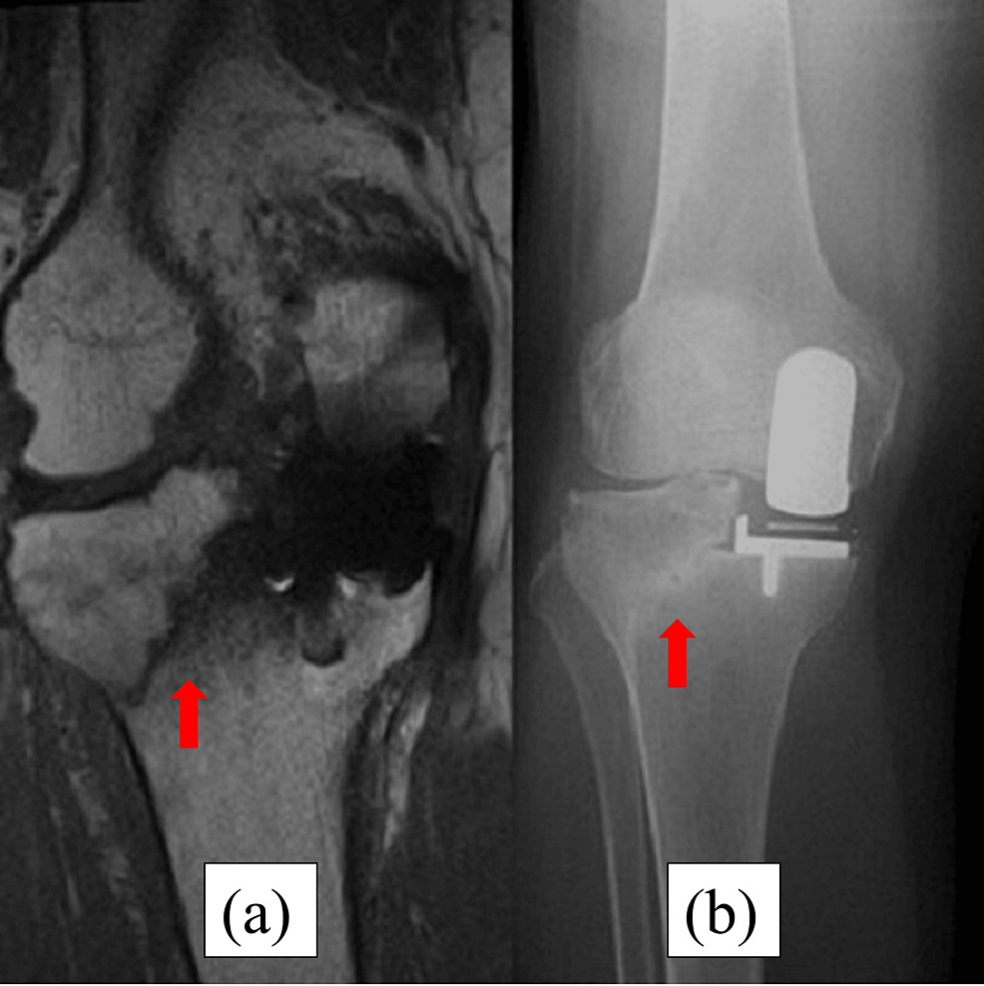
Magnetic resonance imaging (MRI) and radiography at five weeks postoperatively. (a) The red arrow of the MRI shows a fracture line from the intersection of the longitudinal and transverse tibial osteotomies through the lateral pinhole to the end of the lateral tibial diaphysis of the right knee. (b) The red arrow of radiography shows the same fracture line as MRI.
